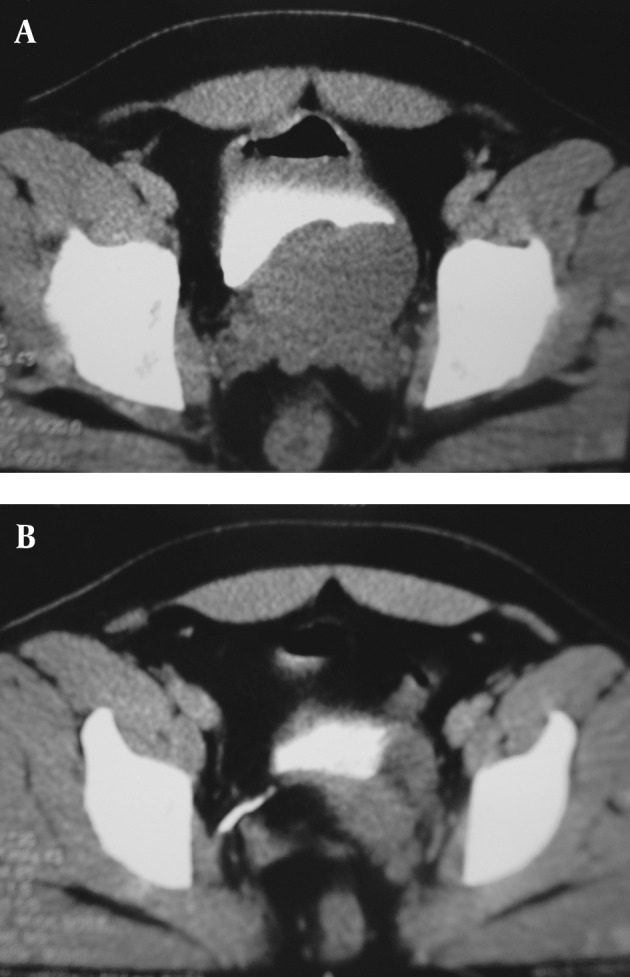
A & B, A 32-year-old man with ureterocele and renal agenesia. There is an external mass effect on the left latero-posterior side of the bladder in CT-cystogram and a normal bladder mucosa.
